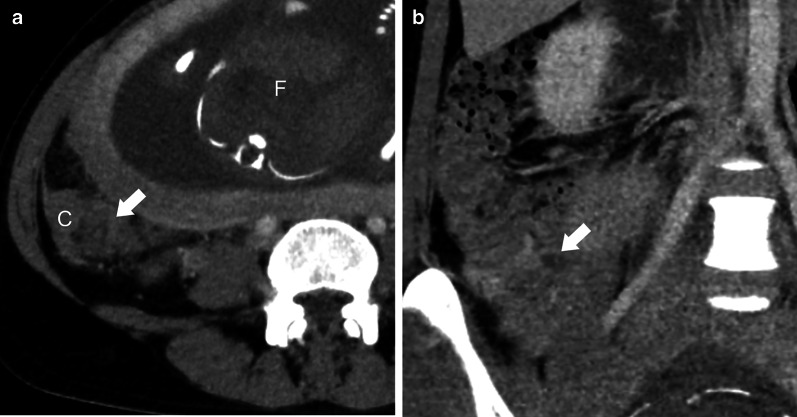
Computed tomography. a Axial view showing increased attenuation in fat (arrow) in the area close to the cecum (C: cecum). ‘F’ indicates the fetus. b Coronal view showing a swollen appendix measuring 10 mm in diameter (arrow) medial to the cecum. c Sagittal view showing that normal appearance of the uterus in pregnancy without findings suggestive of intrauterine infection
